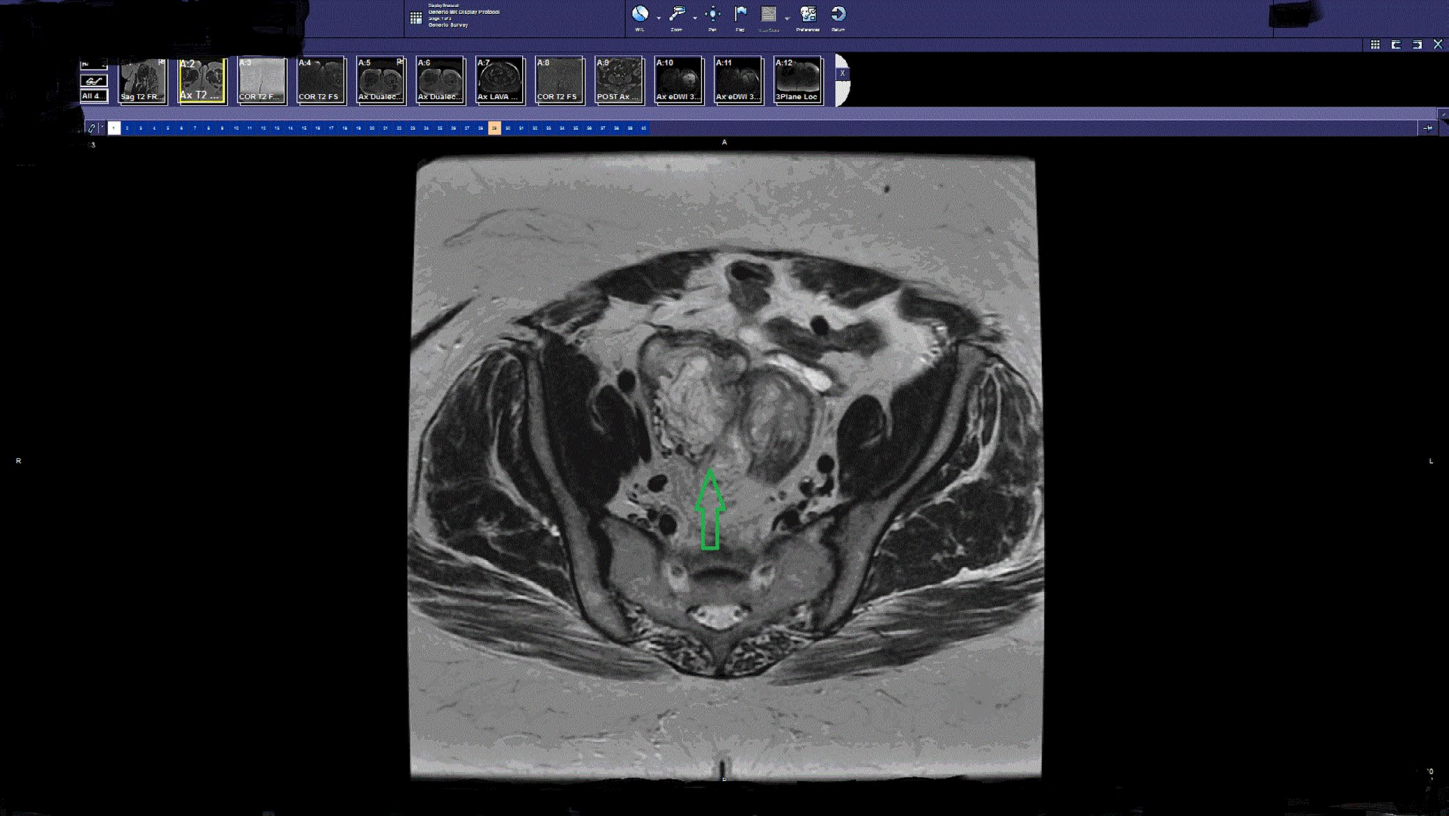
Pelvic MRI image demonstrating the pelvic mass invading into the sigmoid colon. The mass is marked with a green arrow
